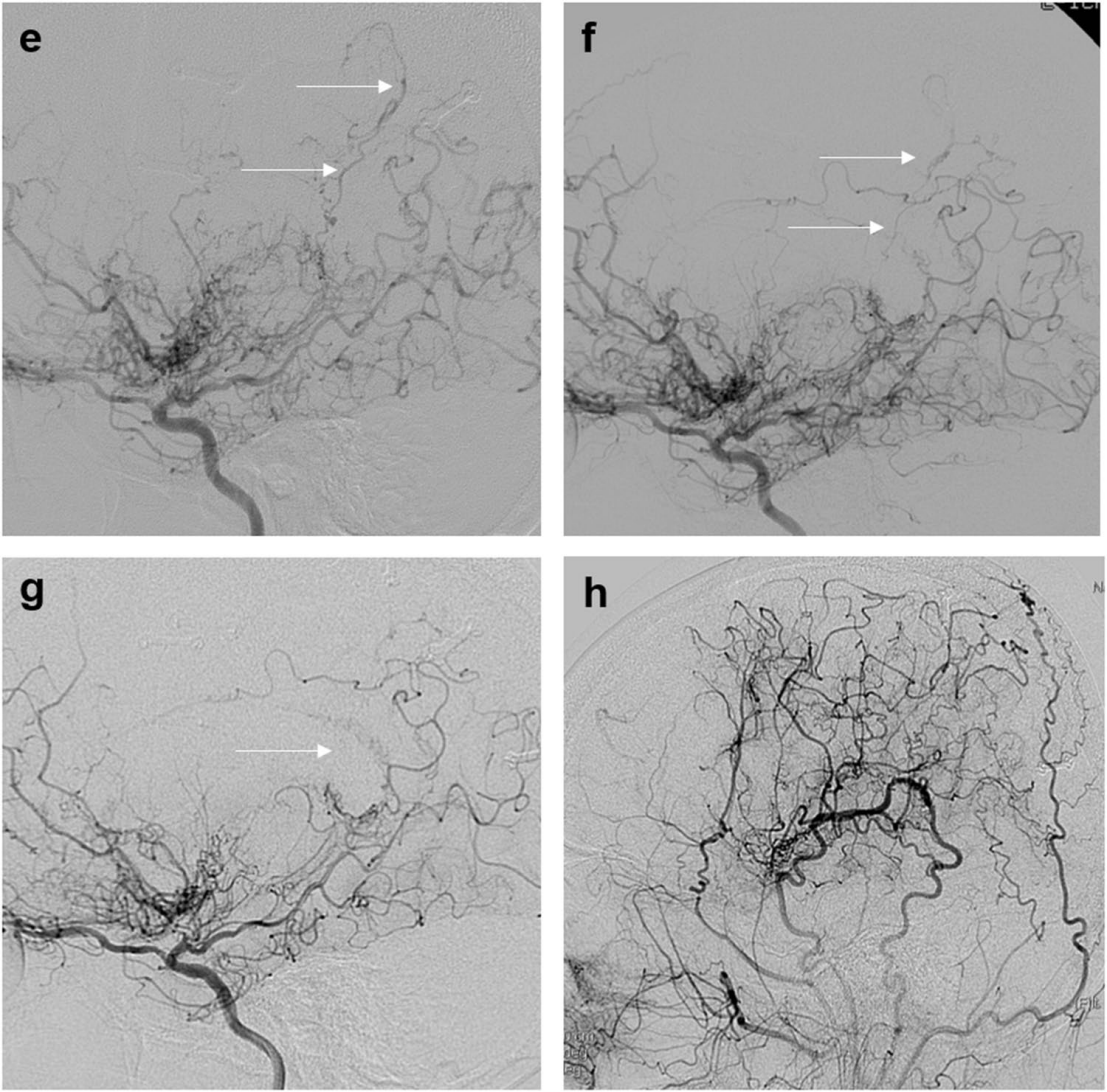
A representative case of periventricular anastomosis in combined and indirect bypass. Lateral view of digital subtraction angiography (DSA) of the vertebral artery in the hemisphere of the indirect bypass demonstrating (a), thalamic anastomosis (white arrow), preoperatively. Two consecutive instances of intracerebral hemorrhage with intraventricular hemorrhage confirmed by brain computed tomography with more prominent thalamic anastomosis (white arrow) in (b), postoperative 2-year and (c), 3-year follow-up DSA. d. Revascularization of indirect bypass in postoperative 3-year right external carotid artery DSA. Lateral view of DSA of the left internal carotid artery revealed choroidal anastomosis (white arrow) in the hemisphere of combined bypass (e), preoperatively and restoration of the choroidal anastomosis (white arrow) at postoperative (f). 6-months and (g). 5-year follow-up. h. Revascularization of combined bypass on postoperative 5-year follow-up DSA
